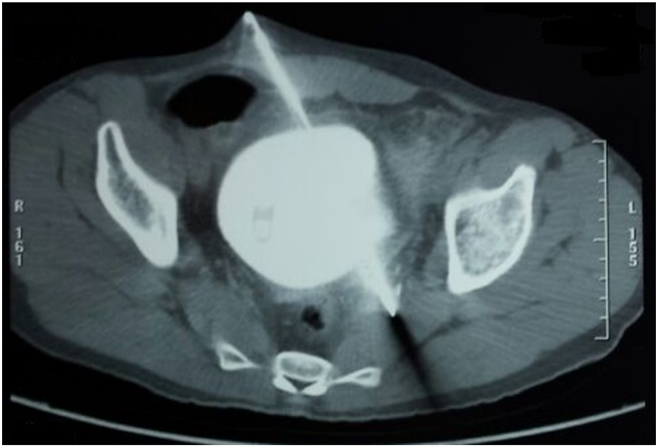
Excretory phase computed tomographic scan showing lesions of the bladder and the left ureter.
